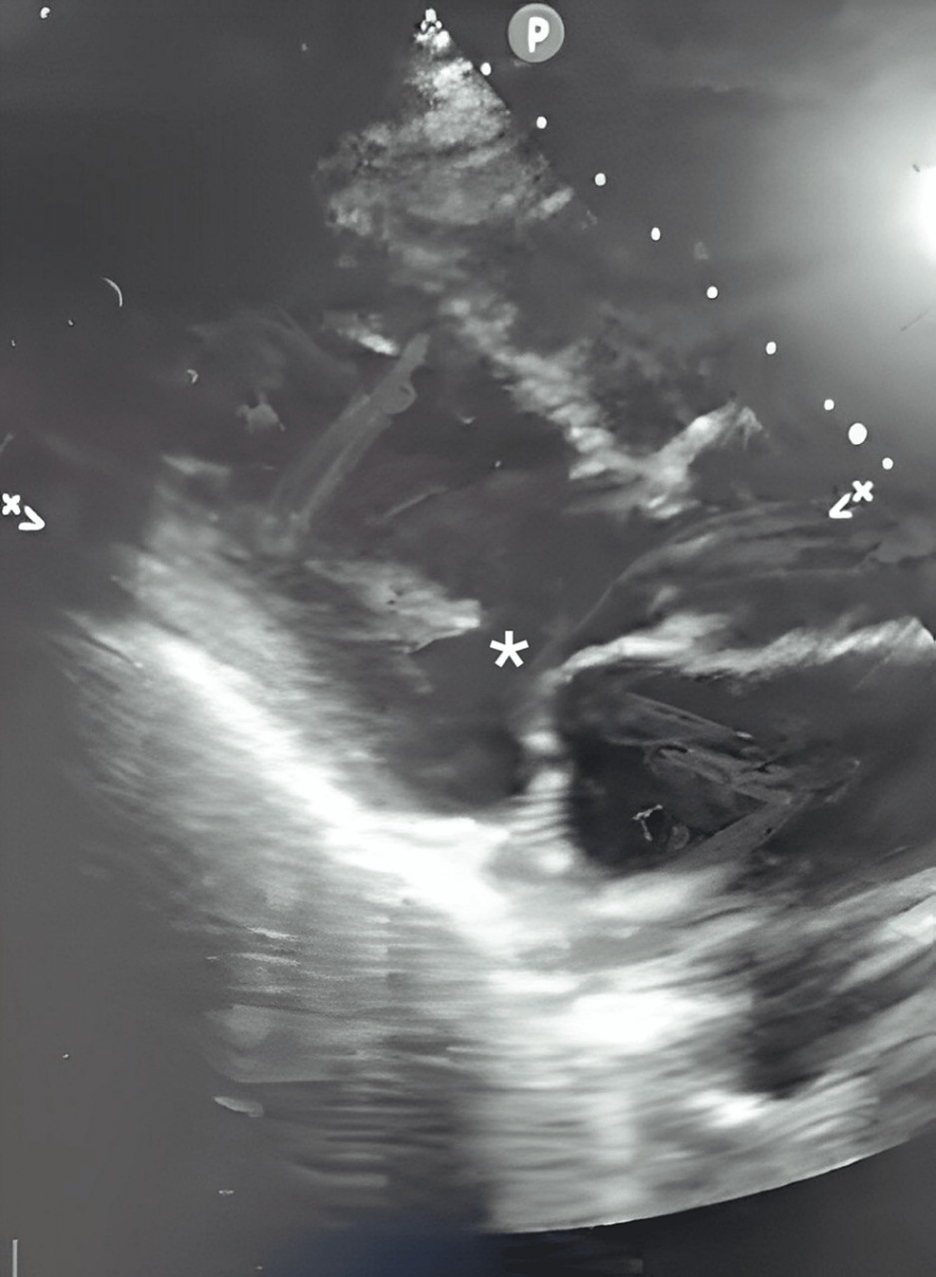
Ultrasound images showing apical ventricular septal rupture * Septal rupture is visible here
